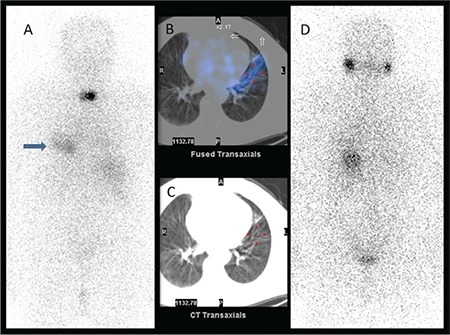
A mild diffuse I-131 uptake at the lower zone of the left hemithorax (arrow) was seen on posterior image of post-therapeutic I-131 whole body scan (A) in addition to residual thyroid tissue. The uptake in the left lung parenchyma was shaped like a thick band that could be compatible with the trajectory of a previous lung infection that was detected on fused single photon emission computed tomography/ computed tomography images (B), and no pathologic finding was found on this area on computed tomography images (C). On diagnostic I-131 scan (D) there wasn’t any pathologic uptake and the serum thyroglobulin level was low (0.7 ng/mL)
